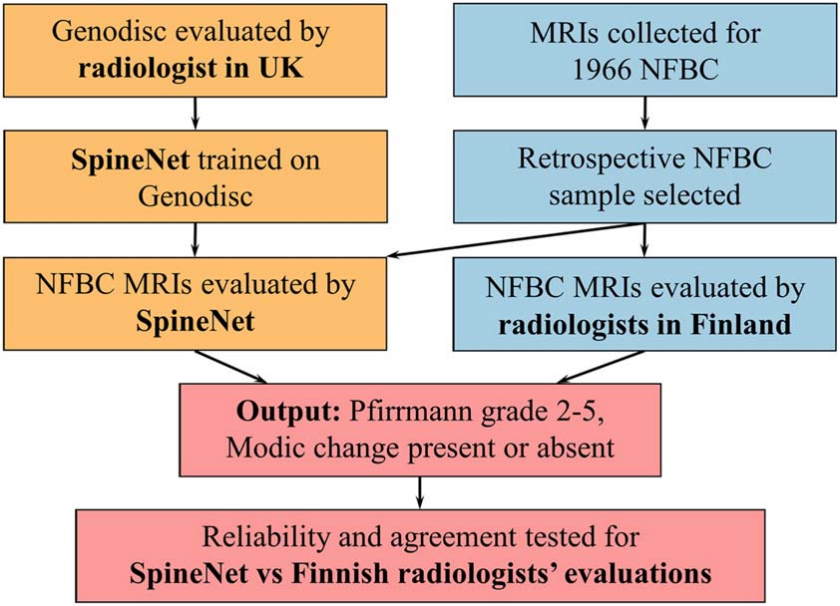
Outline of steps in the external validation process used in this study. MRI indicates magnetic resonance imaging; NFBC, Northern Finland Birth Cohort 1966.
